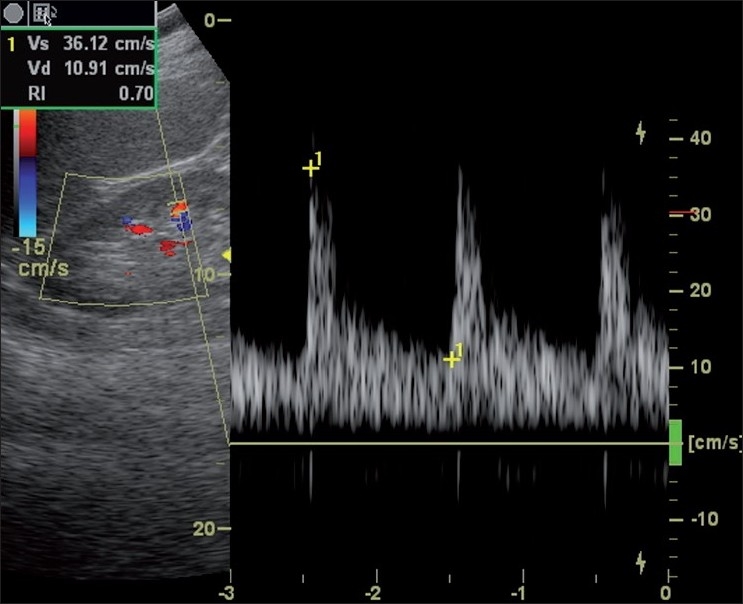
Assessment of intrarenal artery resistive index with patient lying supine using an ultrasound frequency of 3.75 mHz
